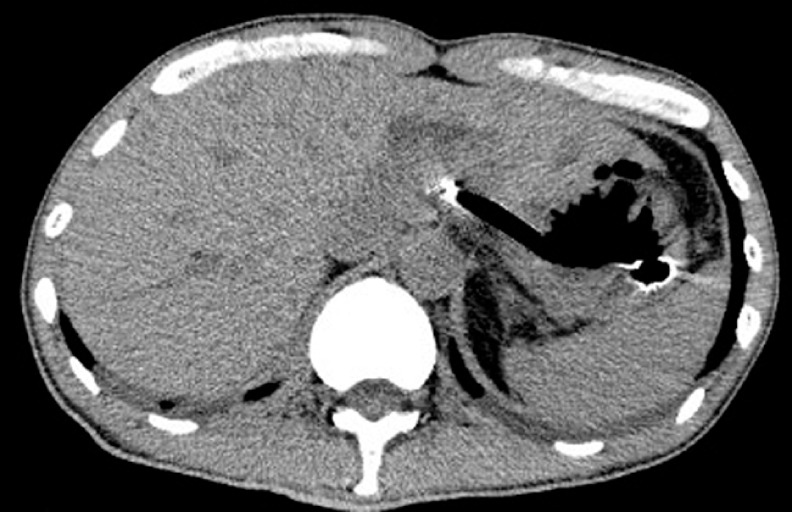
Computed tomography image shows dislocation of an endoscopic ultrasound-guided hepaticogastrostomy (EUS-HGS) stent.
